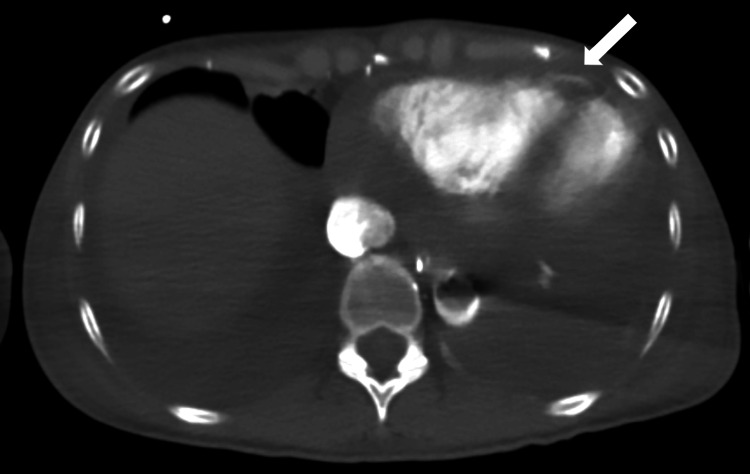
CT angiography. CT angiography showing active contrast leakage from the right ventricle into the pericardial cavity (white arrow), confirming persistent perforation with pericardial hematoma.
